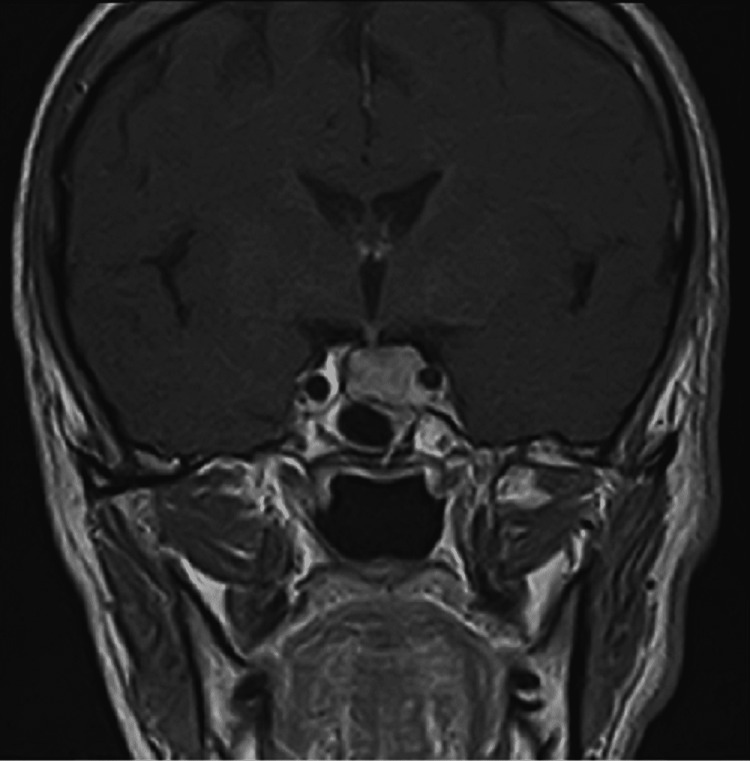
Latest MRI done of coronal cut image showing an isointense pituitary lesion to the grey matter of 1cm x 1.3cm invading the left cavernous sinus with 180 degrees encasement of the cavernous portion of the left carotid artery.
